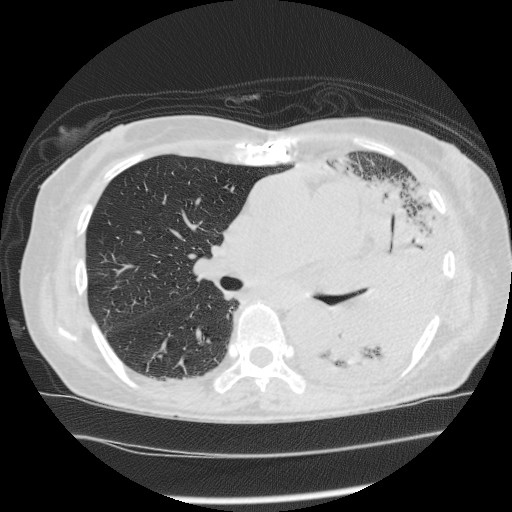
Chest computed tomography on admission revealed consolidation and ground-glass opacity around the left lung.
